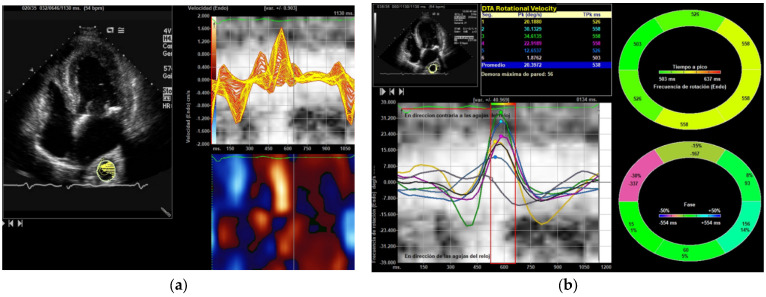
(a,b) DTA rotational velocity is measured using the apical four-chamber view. Parasternal and apical views imaging projections are suitable for Speckle Tracking VVI analysis. The various values throughout the cardiac cycle can be assessed; in this study, we evaluated the average of the distinct systolic peaks.
